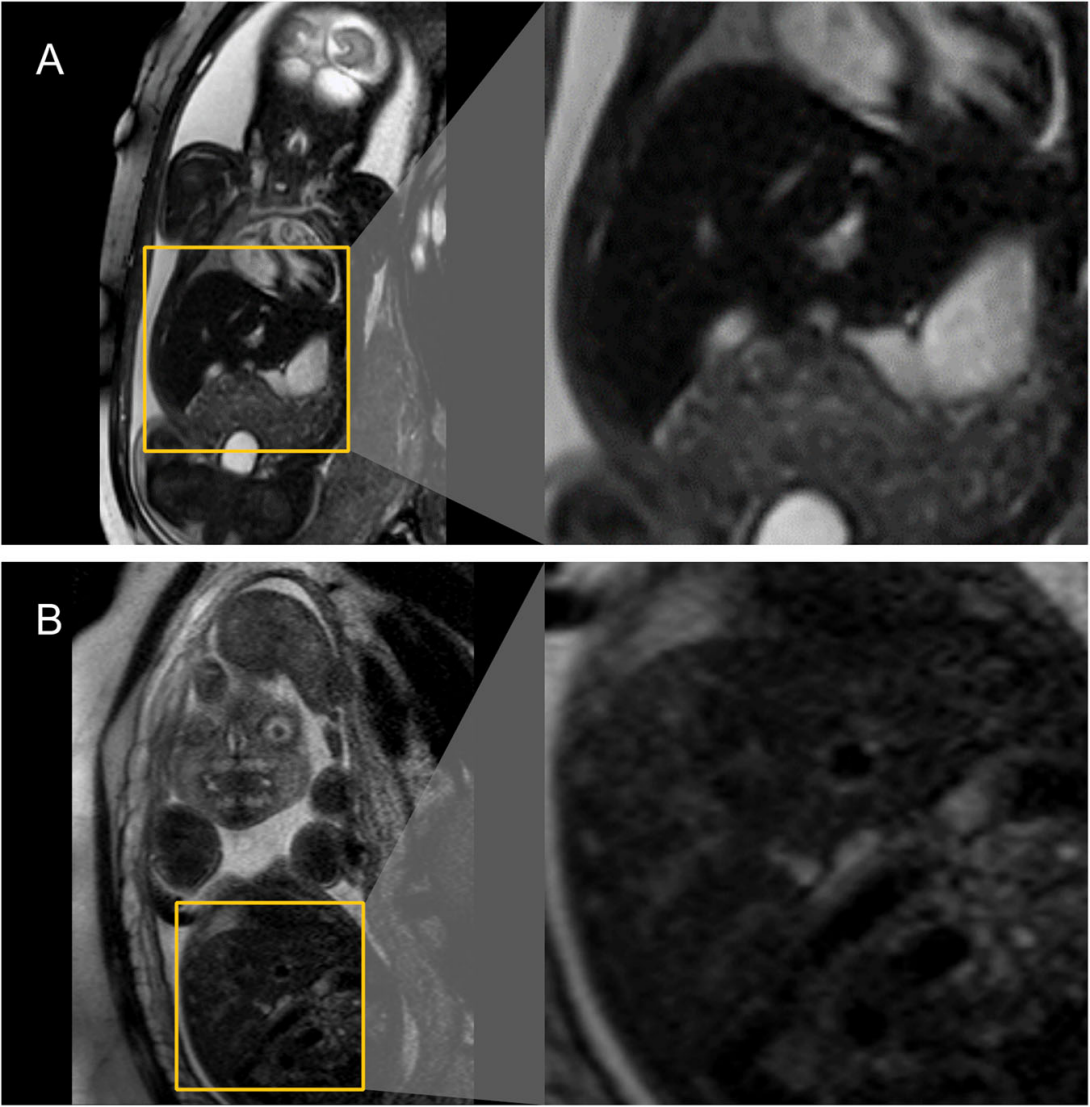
Time-dependent effects of intrauterine transfusions on fetal liver parenchyma on T2-weighted magnetic resonance imaging. A T2-weighted MRI sequence of a fetus at a gestational age of 31 + 1 weeks, who received a total of five IUTs, the last one three days prior to MRI acquisition. On T2-weighted sequences, a profound reduction in the signal intensity of the fetal liver parenchyma is seen (SI = 42). B Images of a different fetus at a gestational age of 28 + 6 weeks, who received one IUT in total, 44 days prior to MRI acquisition. After this time delay of several weeks between IUT and MRI, the SI of the fetal liver steadily increased and no longer shows the profound signal intensity reduction seen in A (SI = 321)
